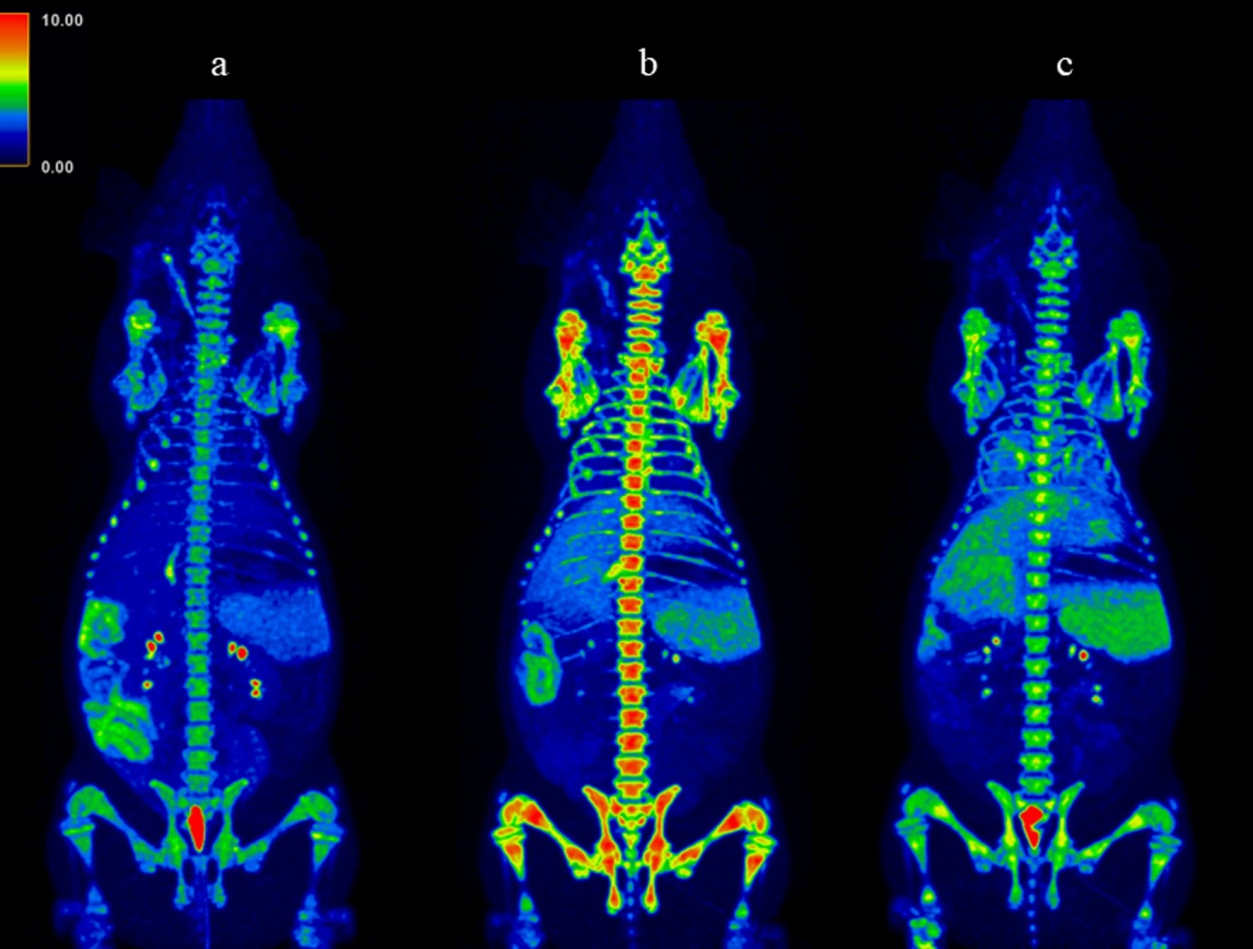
Coronal maximum intensity projection (MIP) PET–CT scans with [^11^C]NES. a − 1 h, b 2 h, c) 4 h. All images are corrected to SUV = 10 and thus directly comparable. The tracer uptake in the bone marrow is significantly increased from baseline − 2 h to 2 h and then decreases. The uptake in the liver is increasing during the progress of sepsis. In the spleen the uptake is increasing at 4 h. We see the elimination of tracer from the kidneys to the urinary bladder and from the biliary tract to the intestine, clearly visible at -2 h, but less distinct after sepsis induction
